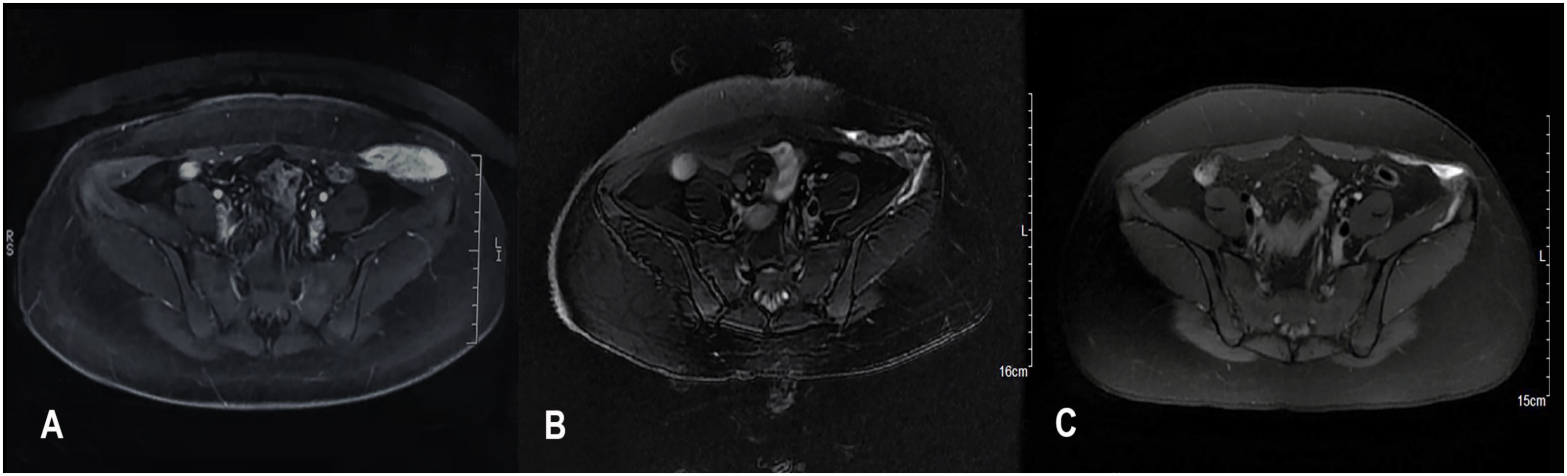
MRI images from before (A) and 3 months after (B) and 6 months after (C) HIFU therapy. (A) A 4.5 cm tumor located at the left lower quadrant; (B) The tumor regressed significantly from 4.5cm to 1.5 cm in diameter with obvious necrosis inside; (C) The tumor was significantly necrotic and absorbed. The MRI sequence is T2 FLAIR.
